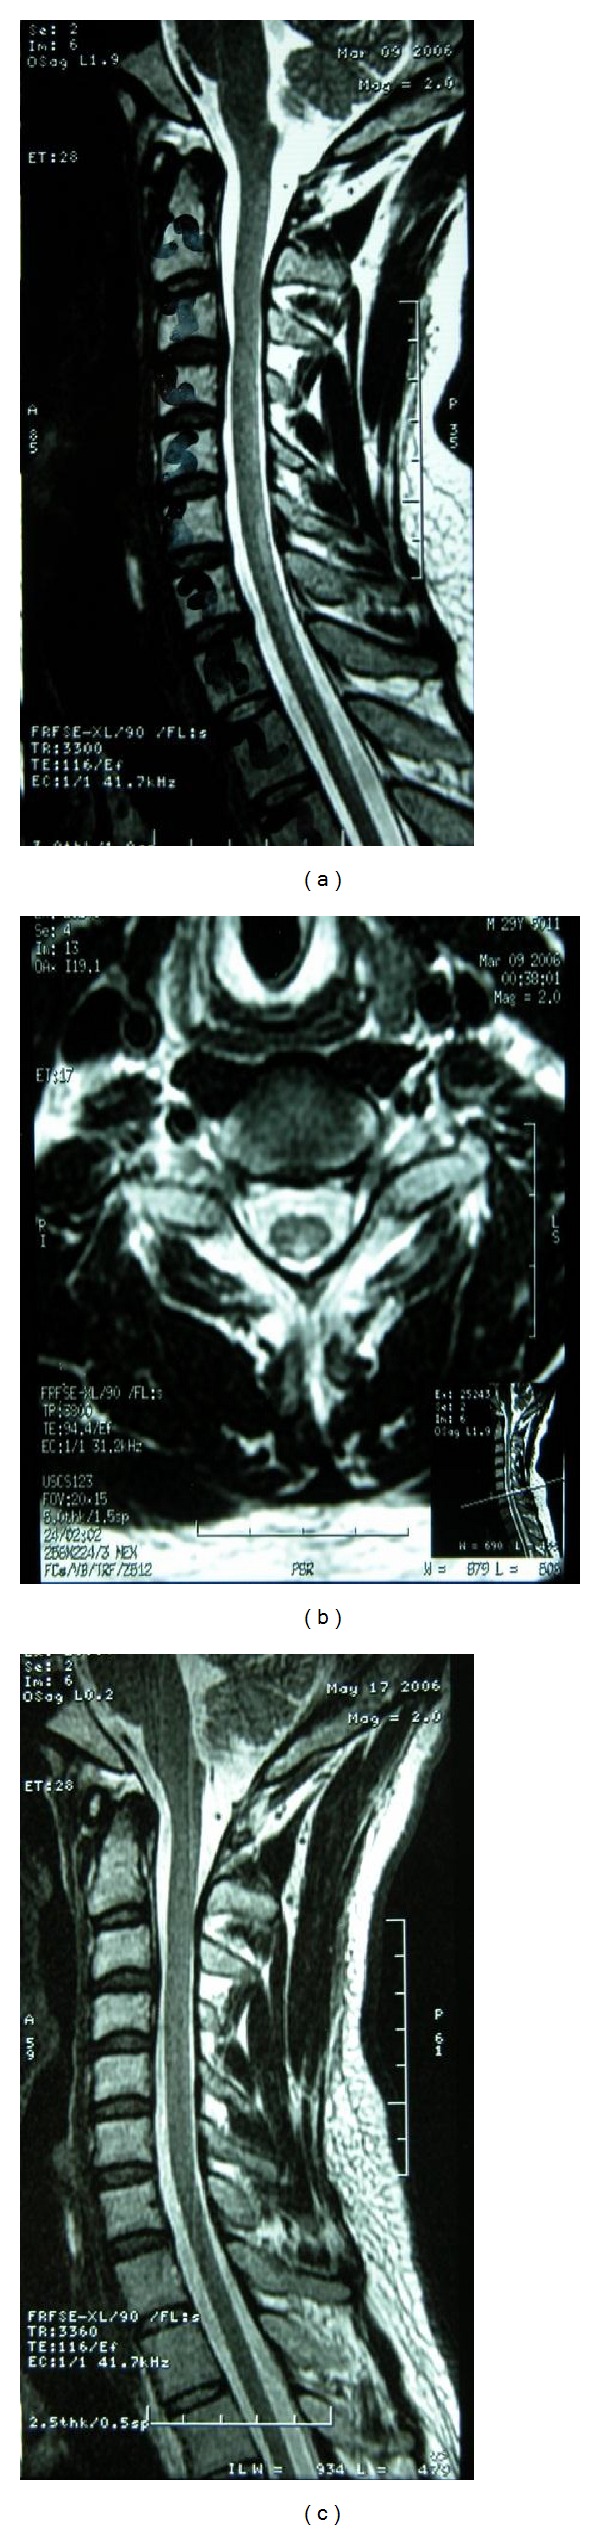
((a), (b)) Sagittal and axial T2 magnetic resonance images on patient admission showed T2 hyperintensity of the posterior columns. (c) Sagittal T2 magnetic resonance images 2 months later showed striking reduction of the MRI abnormalities.
